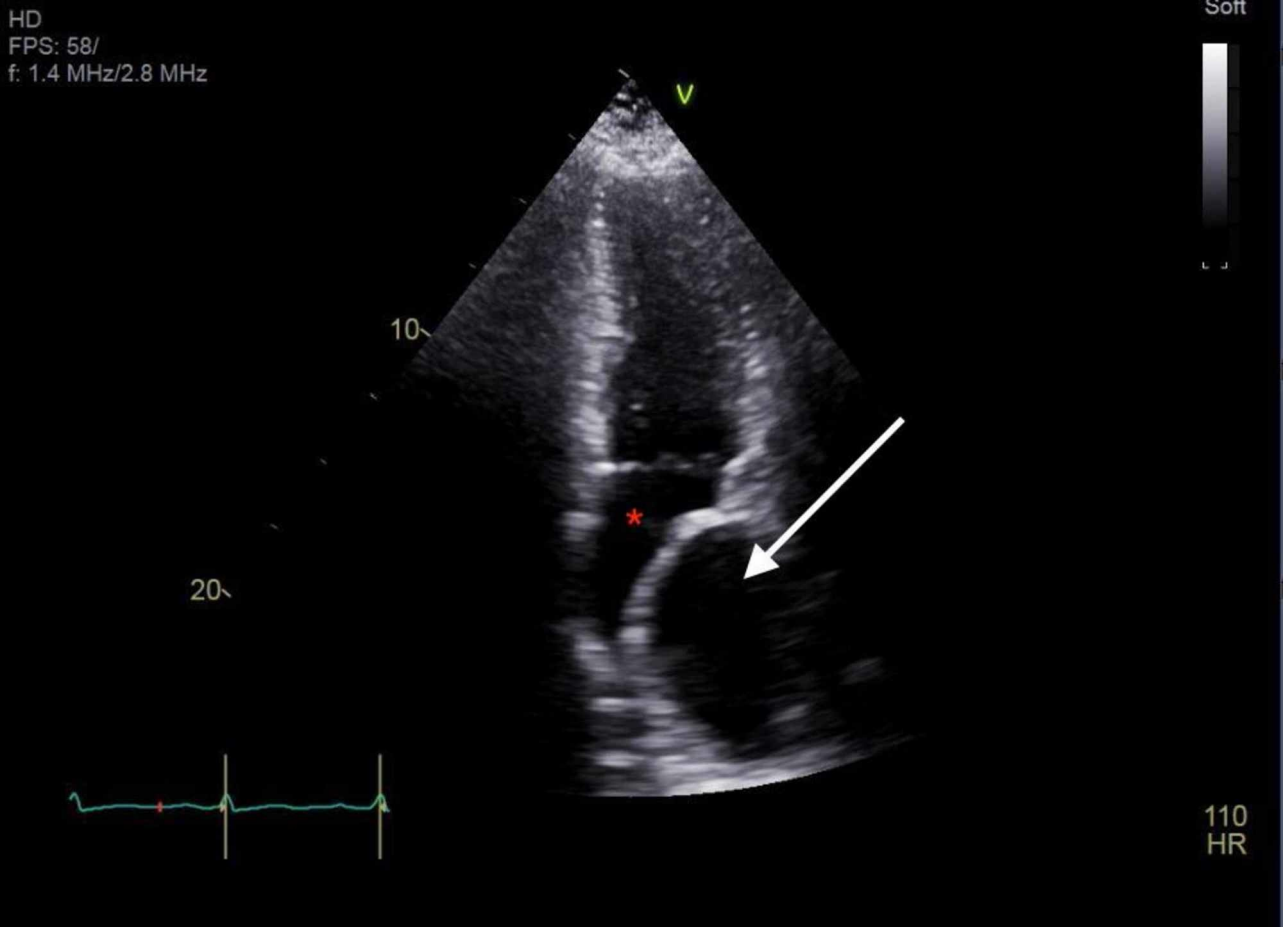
Transthoracic echocardiogram showing cyst-like structure (white arrow) compressing the left atrium (red asterisk).
